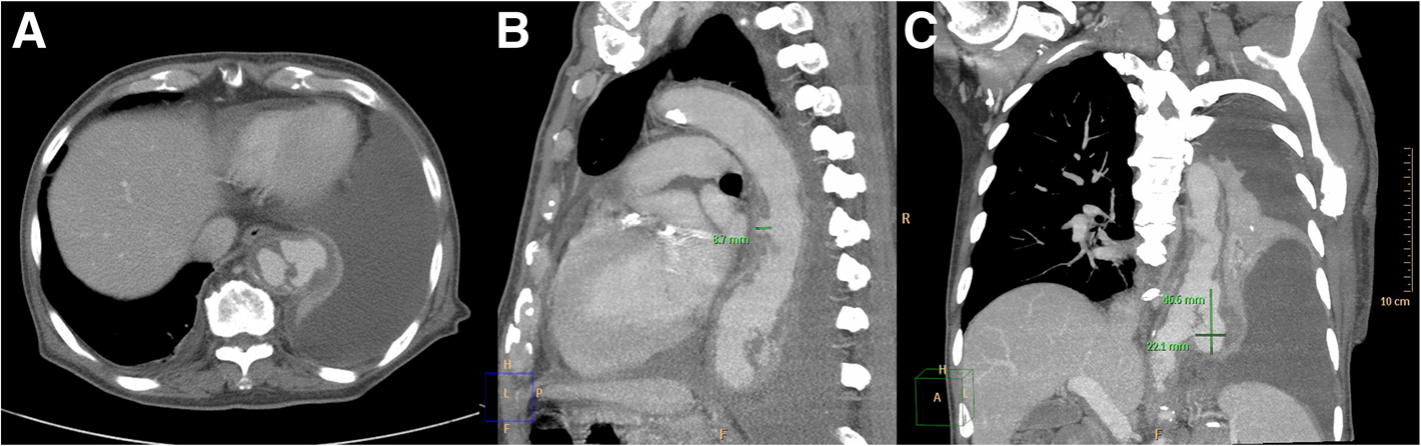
Axial, sagittal and coronal views of computed tomographic scan with intravenous contrast media of chest showed irregular dumbbell-shaped aortic lesion (Panel a), multiple mushroom-like thoracic aortic aneurysms with the size of 8.7 mm in diameter (Panel b) and 46.6 mm × 22.1 mm (Panel c) with periaortic soft tissue density at the level of between 8th and 11th thoracic spine, massive pleural effusion and atelectasis of left lung
